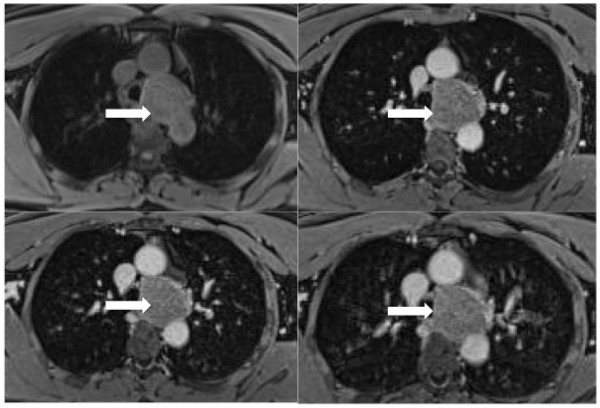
T1-weighted volumetric interpolated breath-hold examination (VIBE) sequences on axial planes. The post-contrast T1-weighted images show poor enhancement within the solid lesion (white arrows).
